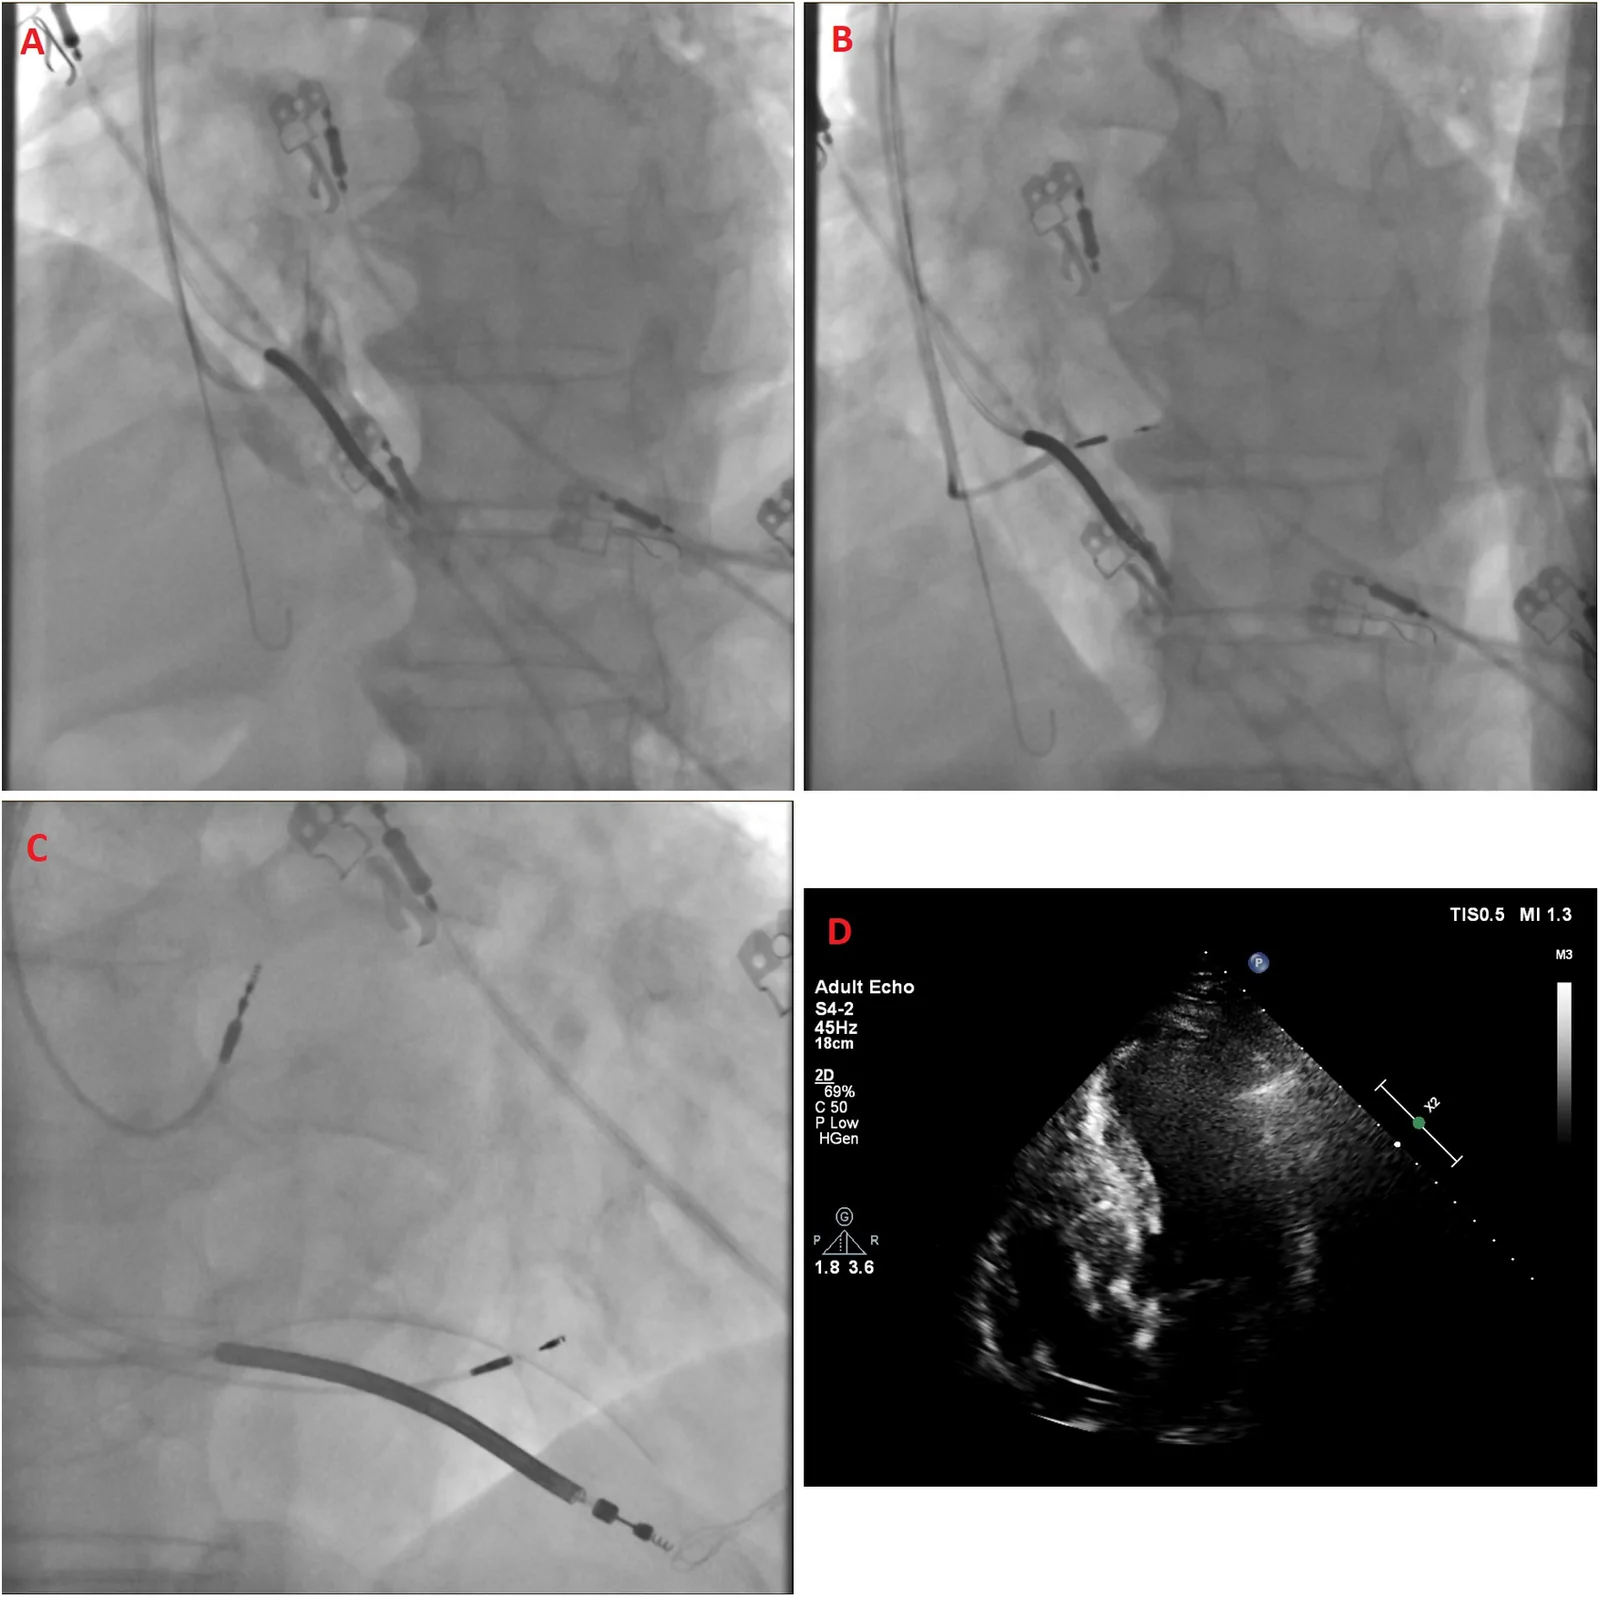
Fluoroscopic and echocardiographic assessment of septal lead implantation. (A) LAO 30° fluoroscopic projection demonstrating sheath positioning at the intended septal implantation site before lead advancement. (B) Contrast-enhanced fluoroscopic imaging demonstrating apparent intraseptal lead advancement. (C) RAO 30° fluoroscopic projection demonstrating the final lead trajectory and position. (D) Apical four-chamber echocardiographic view demonstrating stable intraseptal lead position within the markedly hypertrophied interventricular septum.
